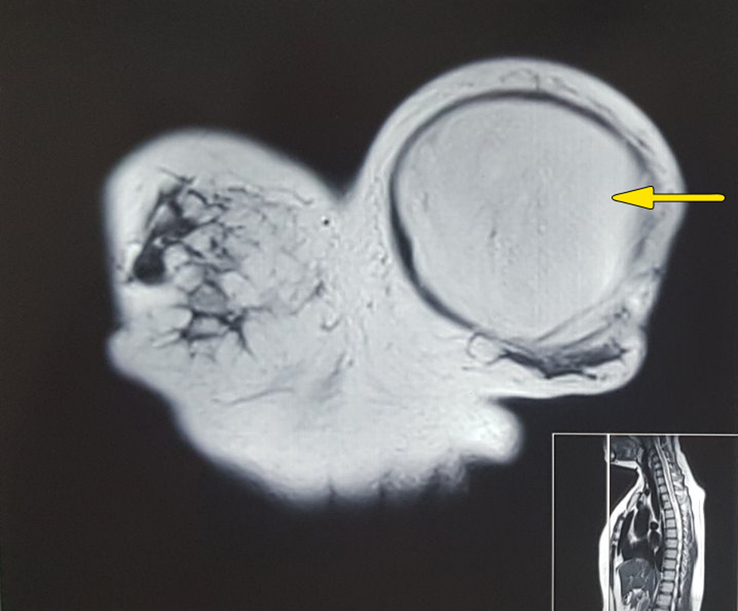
Magnetic resonance imaging showing well circumscribed homogeneous T2 high signal intensity lesion in the left breast (arrow); the lesion showed high signal intensity in T1 with suppression in STIR (not shown here).
